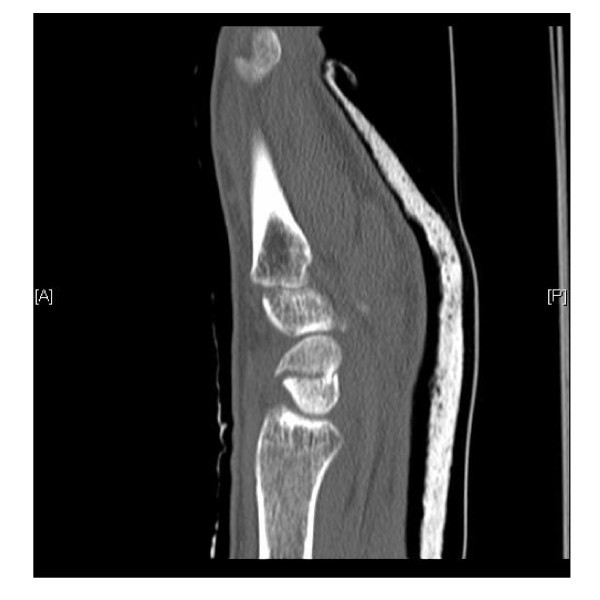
CT scan (patient B) sagittal view of a fractured scaphoid.
